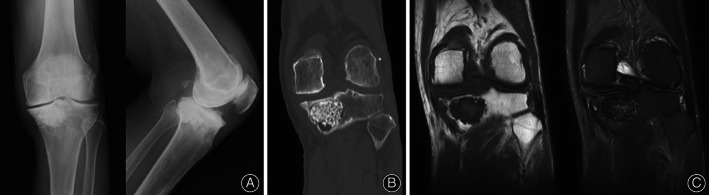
(A) A simple knee joint X‐ray showed degenerative changes in the medial aspect of the left knee, indicating Kellgren–Lawrence grade 4 knee osteoarthritis. There was also a mass lesion with decreased permeability within the proximal tibia bone, but there were no obvious malignant findings such as periosteal reaction. (B) Left knee CT showed a left proximal tibial mass with localized, well‐defined margins and internal granular calcification. (C) Left knee MRI showed a left proximal tibial mass with low‐signal granules and clear margins in both T1 and T2
